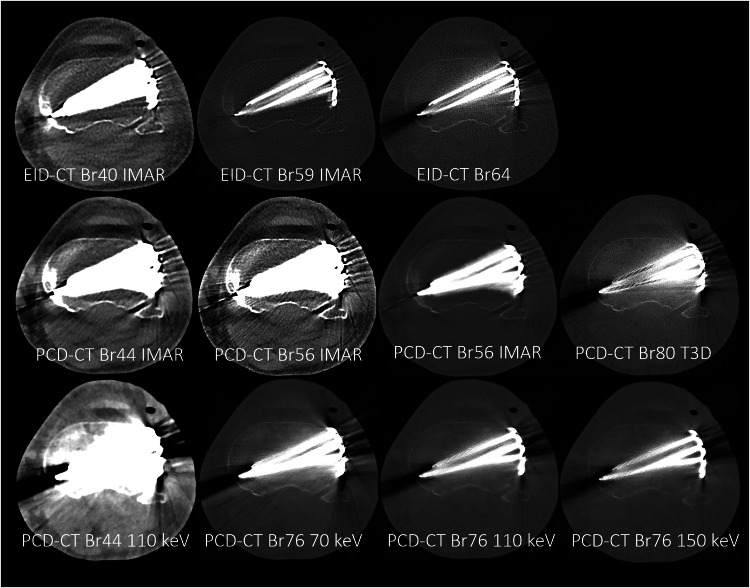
Representative axial CT images of a fixation plate and screws at the proximal tibia. iMAR reconstruction markedly reduces streak artifacts, though small hyperdense areas remain adjacent to screws. The top row displays EID-CT images, while the middle and bottom rows show PCD-CT images. Window settings are optimized for the intended use, with soft-tissue evaluation shown on the left and bone evaluation on the right
